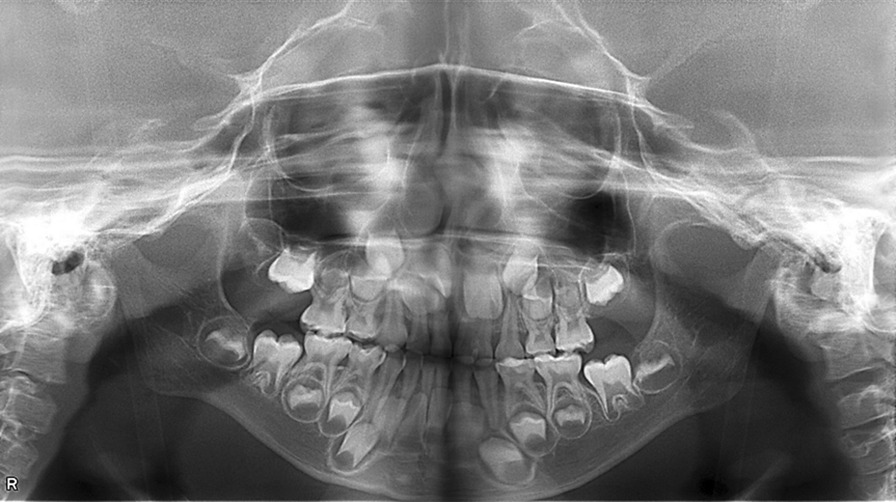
Panoramic radiograph showing the absence of the maxillary left second molar and lateral incisor
